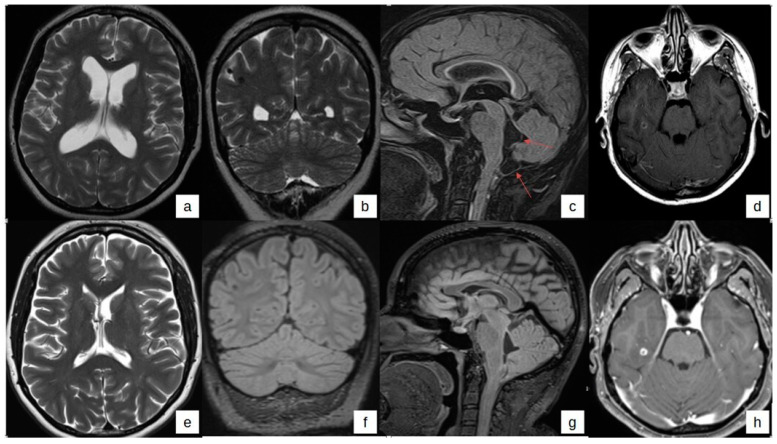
Brain MR imaging at diagnosis (a–d) and follow-up (e–h). (a–d). MR images obtained in 2020 using T2-weighted axial, T2-weighted coronal, T1-FLAIR sagittal with contrast, and T1-weighted with contrast axial sequences. Findings include dilatation of the supratentorial ventricular system (a), subcortical localization in the right parietal region (b), pachymeningeal enhancement observed in the dilated cisterna magna and cervical spine (c) (the red arrows indicate cysts in the fourth ventricle and cisterna magna), and a small subcortical localization with ring enhancement in the right temporal region (d–h). MR images obtained in 2023 using T2-weighted axial, T2-weighted coronal, T1-FLAIR sagittal with contrast, and T1-weighted with contrast axial sequences. Notable changes include regression of ventricular dilatation (e), persistent subcortical localization in the right parietal region (b), collapse of the cisterna magna with reduced pachymeningeal enhancement (g), and unchanged subcortical localization in the right temporal region with ring enhancement (h).
